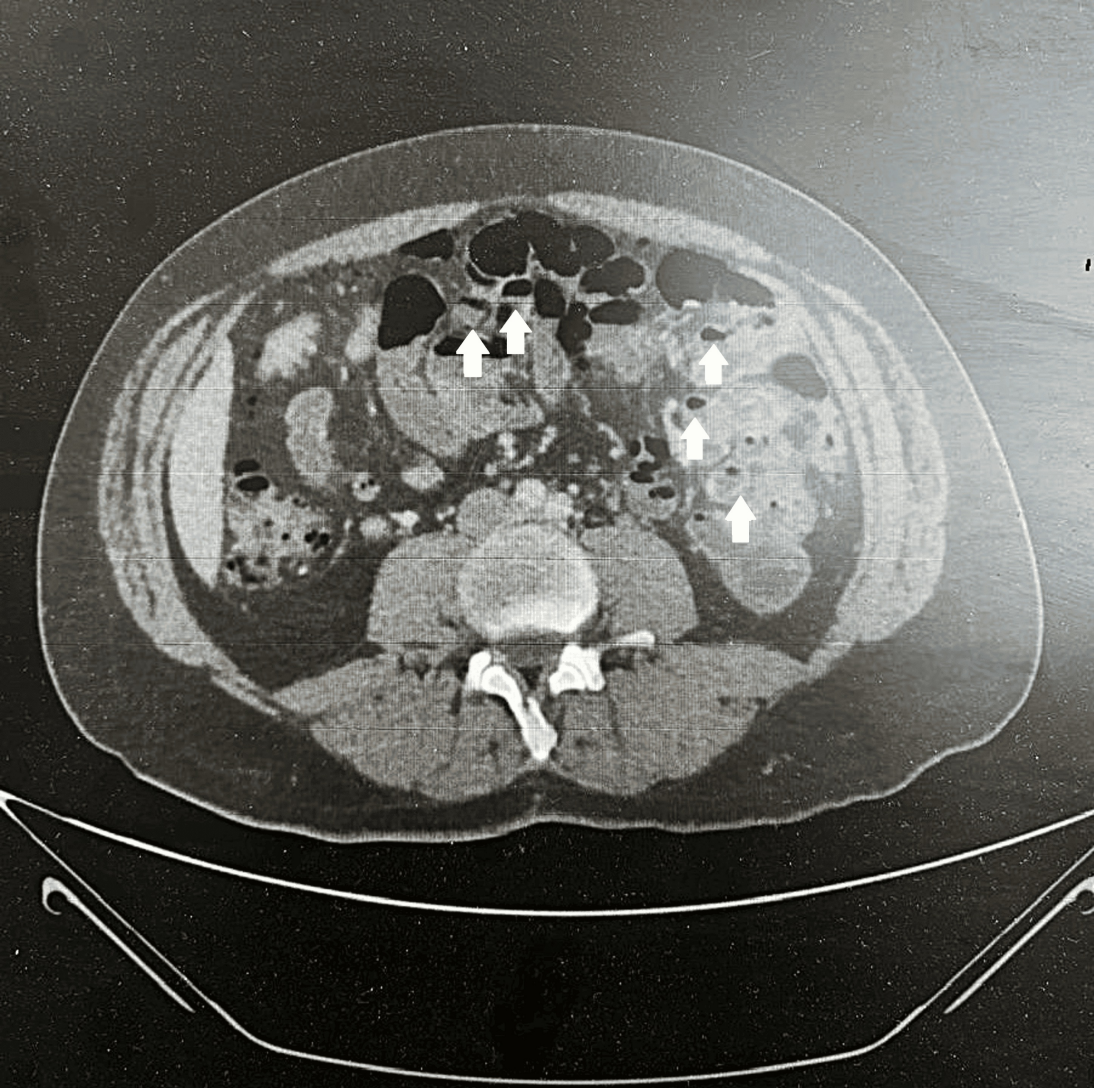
Transverse abdominal CT scan with IV contrast showing multiple small bowel diverticula with pneumatosis.
